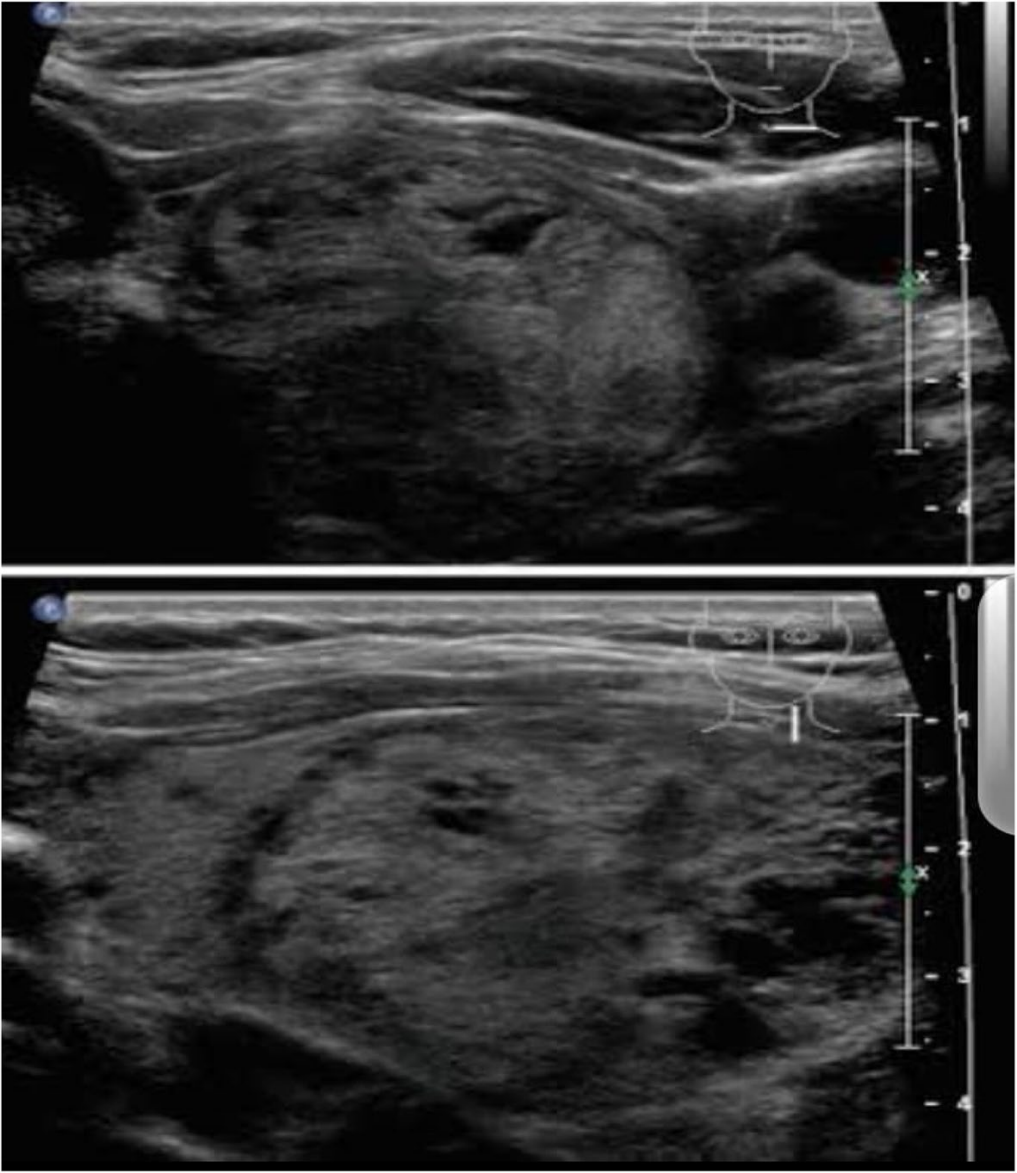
Gray-scale ultrasound images show a right thyroid nodule described as TIRADS IV in a Group I participant
